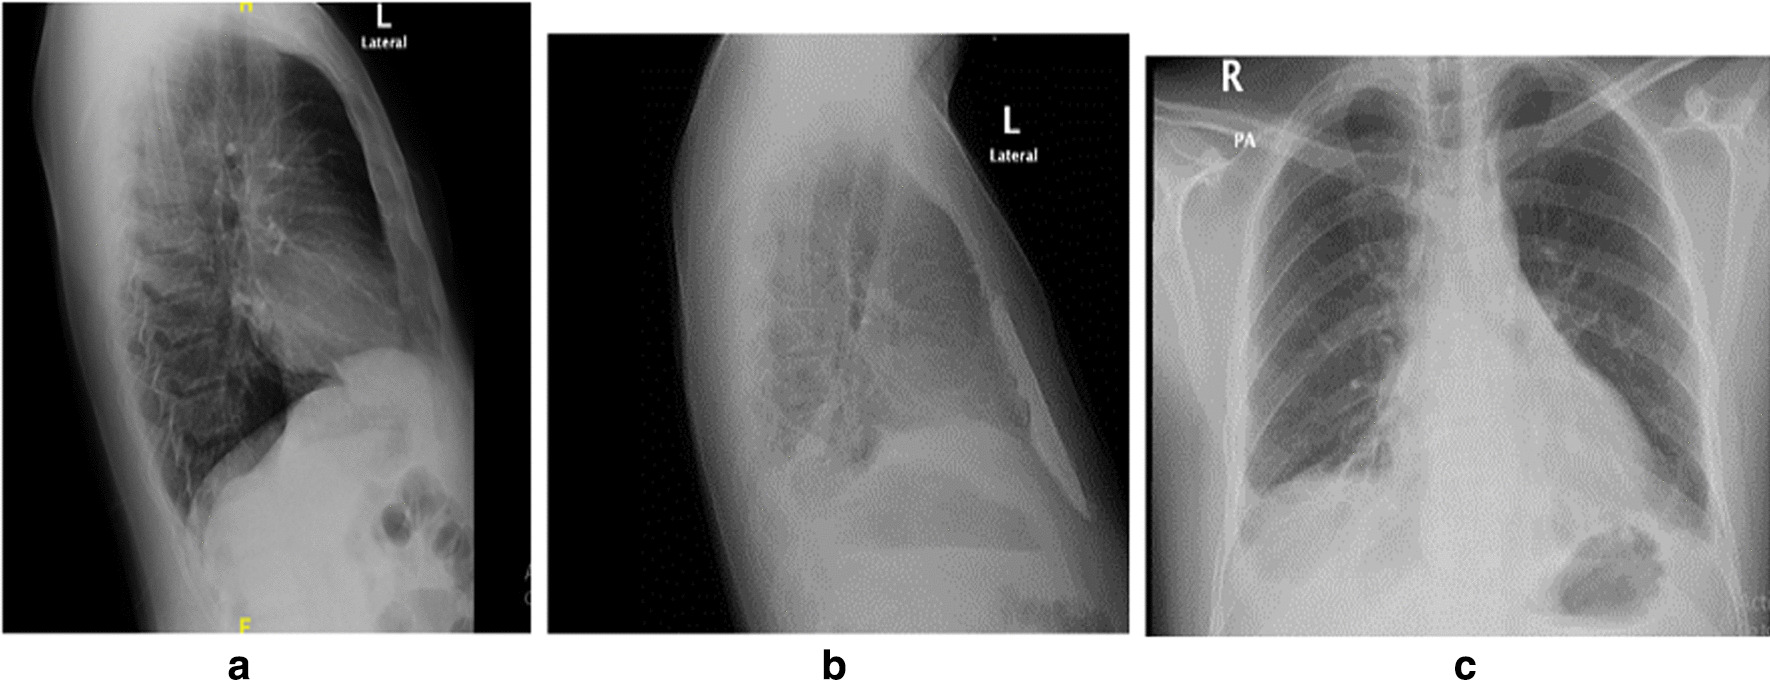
a Chest radiograph lateral view: showed swelling at the lower part of the sternal body. b Chest radiograph lateral view: showed synthetic material at the lower part of the sternal body. c Chest radiograph posteroanterior view: showed blunted both costophrenic angles
